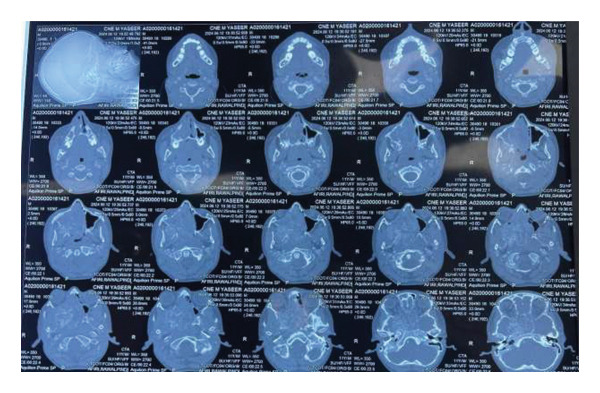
CT PNS with contrast axial view: A large enhancing, highly vascular soft tissue lesion is seen in the right posterior choana, extending into the nasopharynx and nasal cavity. The lesion infiltrates adjacent structures, including the right maxillary sinus, causing expansion and remodeling of the bony walls.
